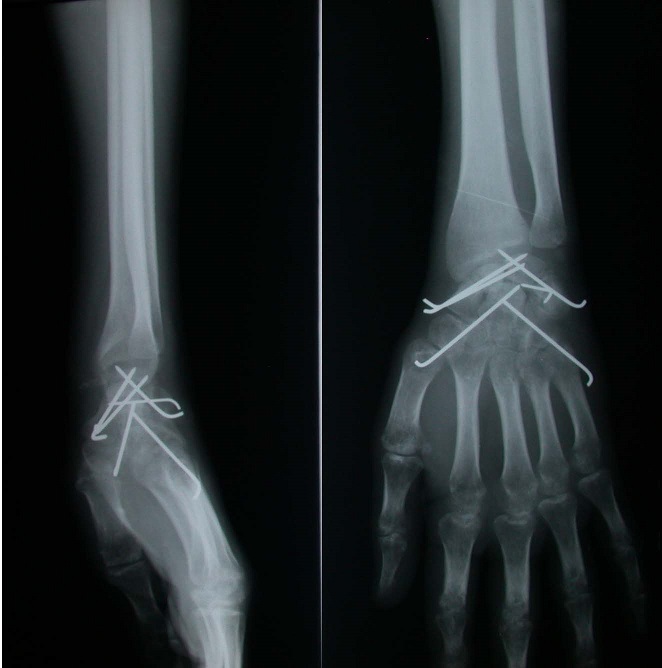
Radiographies de contrôle après embrochage des os du carpe
